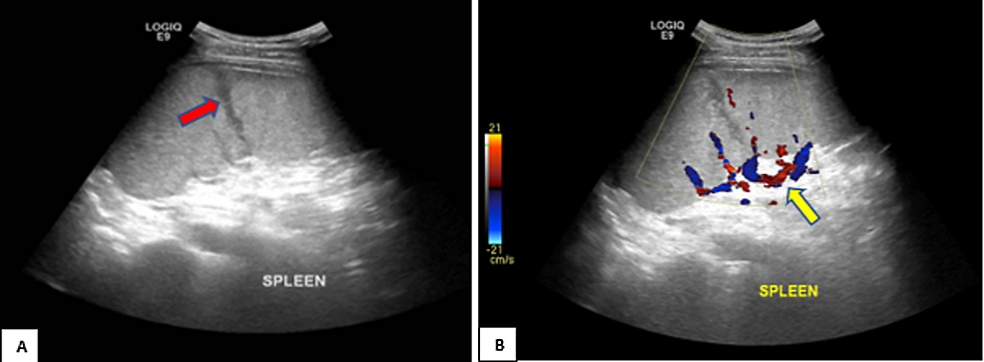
Ultrasound abdomen showing A) focal hypoechoic linear area in the spleen extending from the cortex (red arrow) and B) no vascularity on Doppler imaging (yellow arrow)
